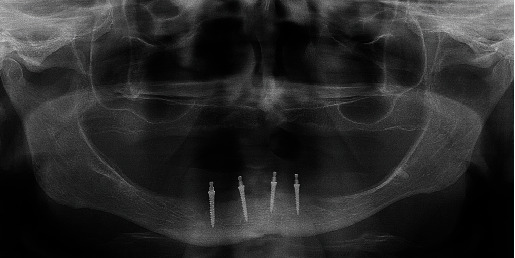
The control panoramic radiogram 1 year after loading of mini-implants.
